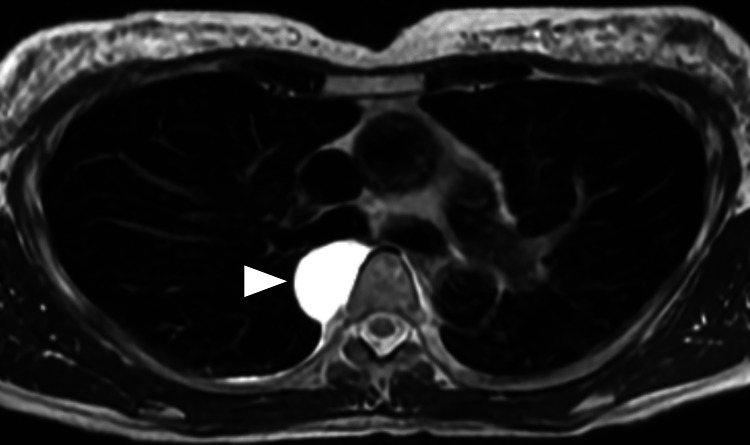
Chest magnetic resonance imaging (MRI) T2-weighted MRI showing a hyperintense lesion suggestive of a cystic nature (arrowhead).
